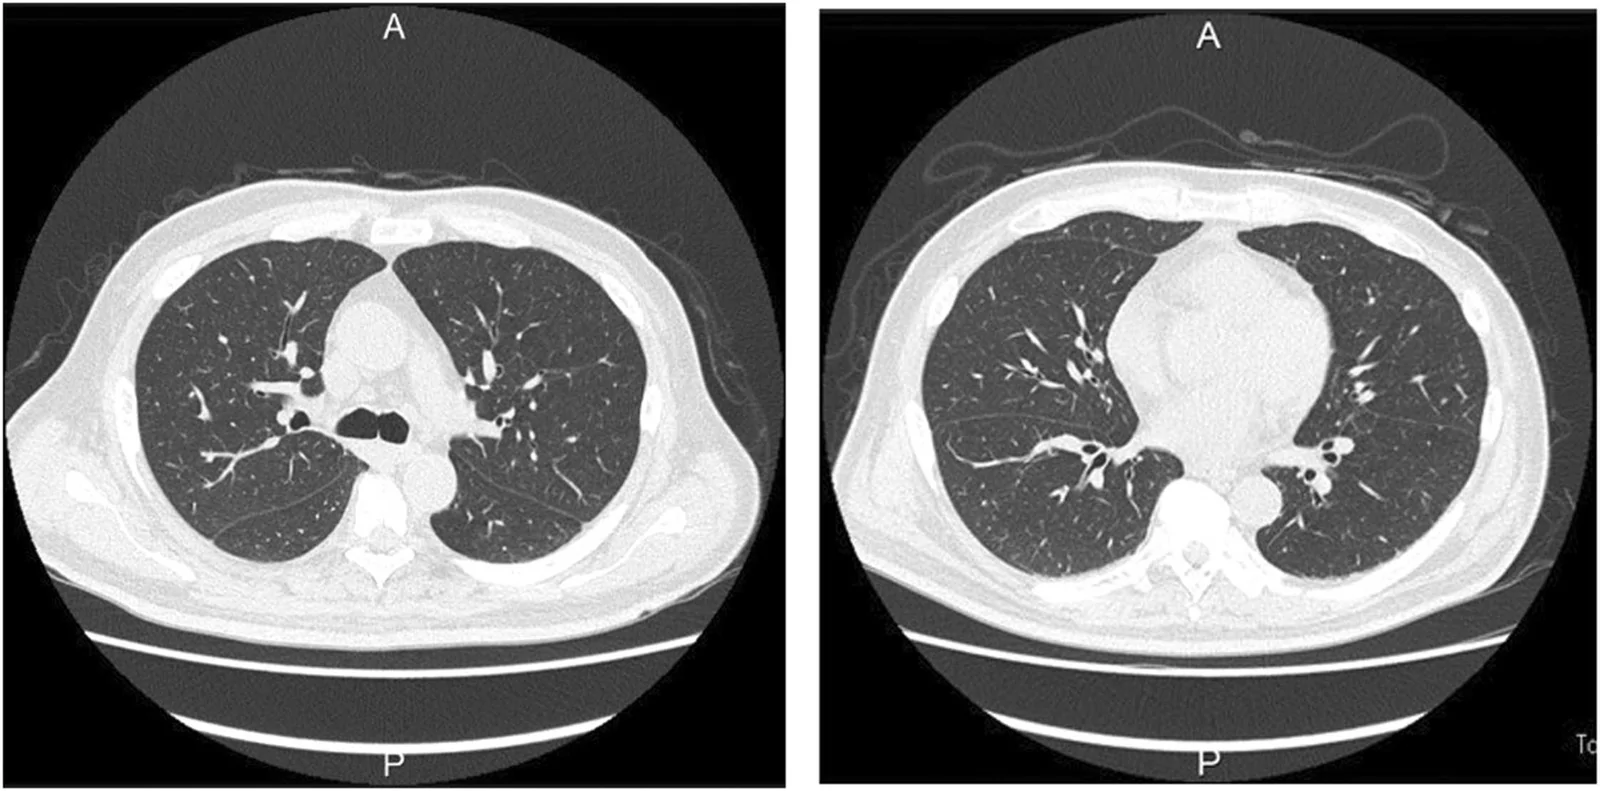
Chest computed tomography 18 days after starting intravenous acyclovir revealed that the lesions had almost disappeared.
